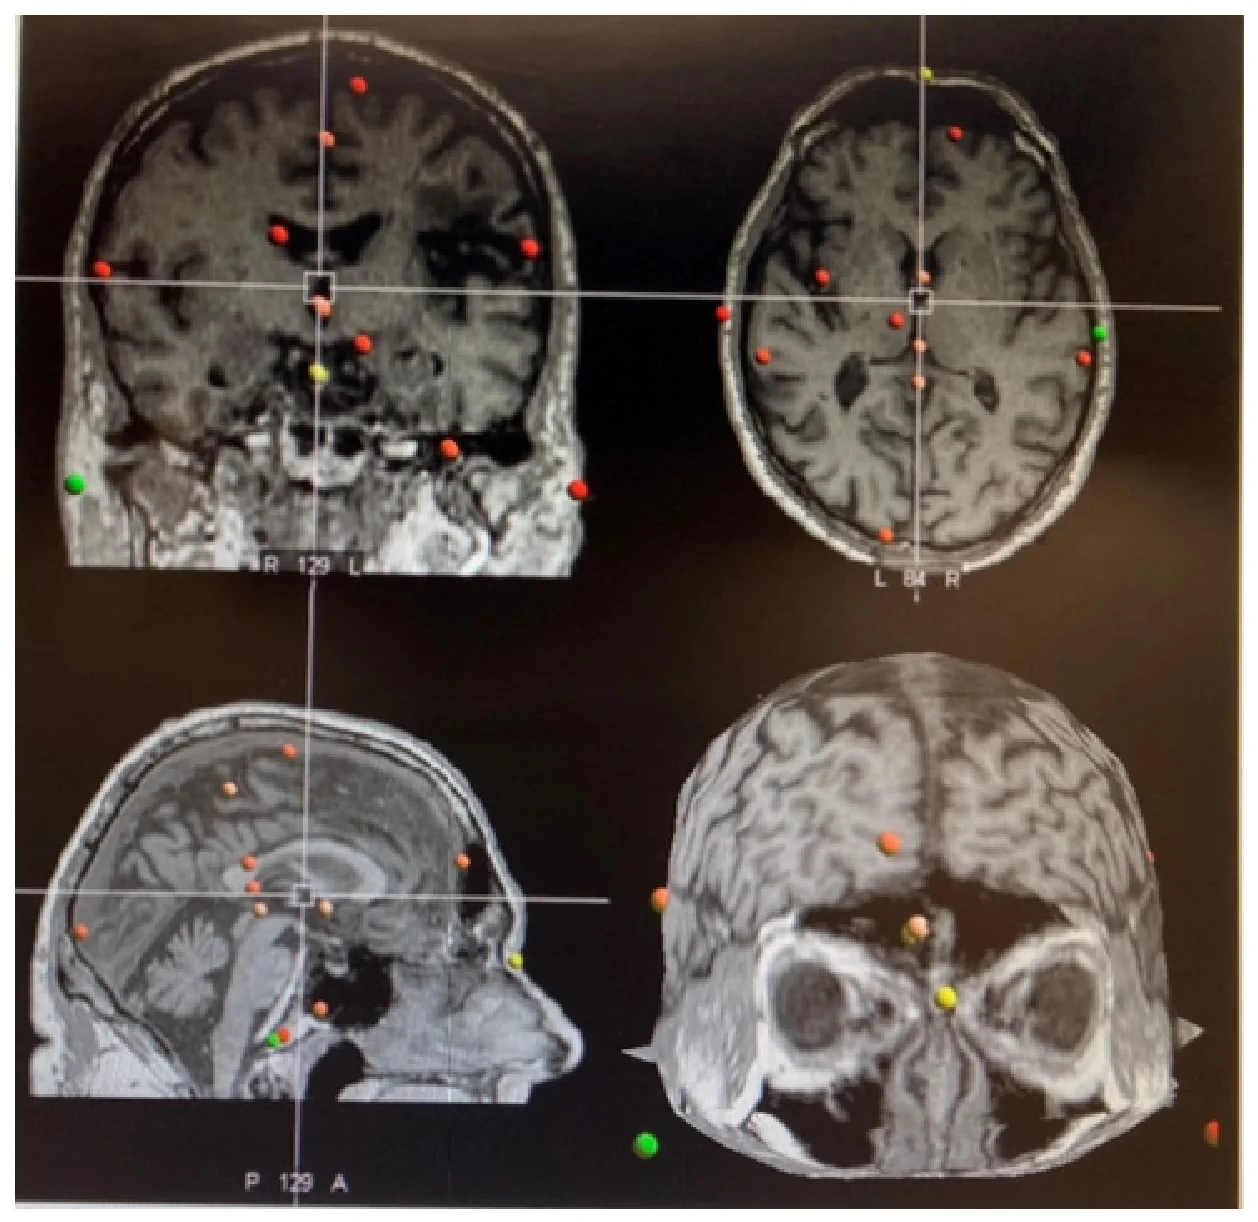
Brain MRI of PA2 six weeks before therapy.
